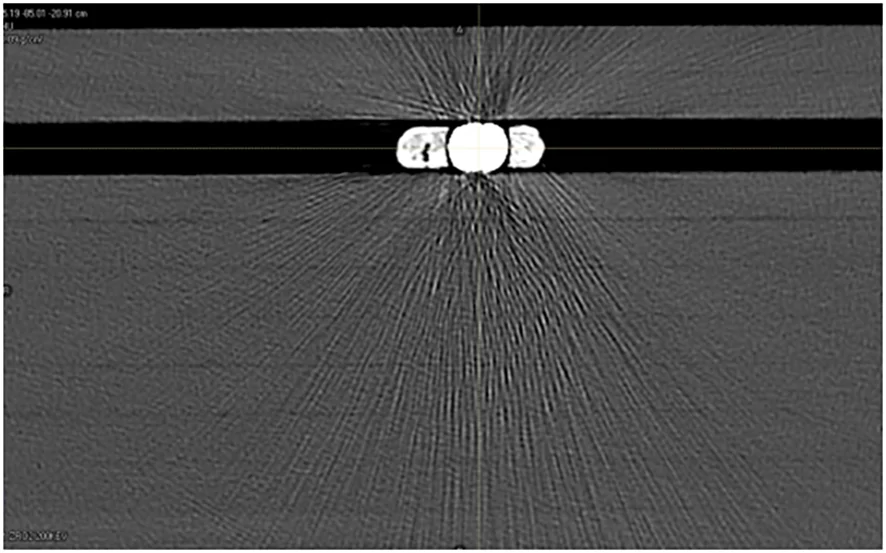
De-artifact CT image (ZrO_2_).
